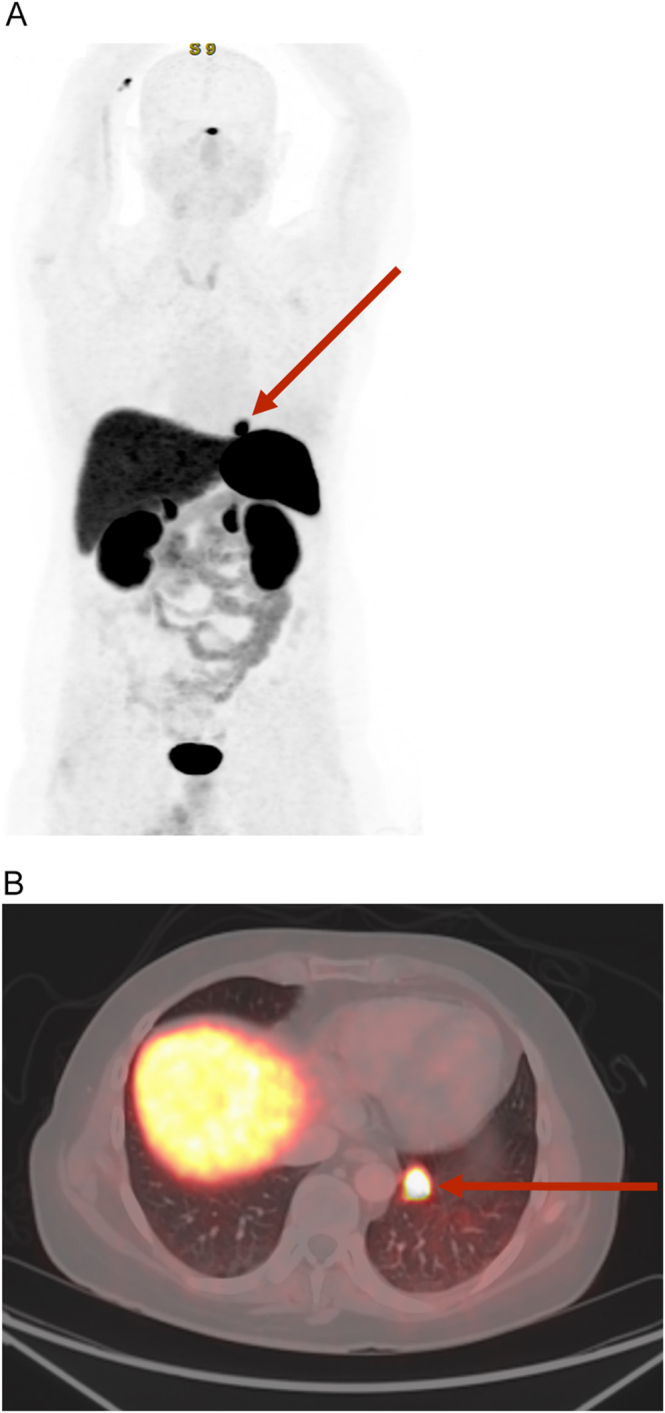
^68^Ga-DOTATOC-PET-CT scan. (A) Maximum intensity projection (MIP) PET image showing focal uptake in the left lower lobe (LII) (arrow). (B) Fused PET/CT image showing high uptake in the pulmonary nodule in the LII (SUVmax of 21.92, Krenning score of 3), consistent with somatostatin receptor overexpression.
